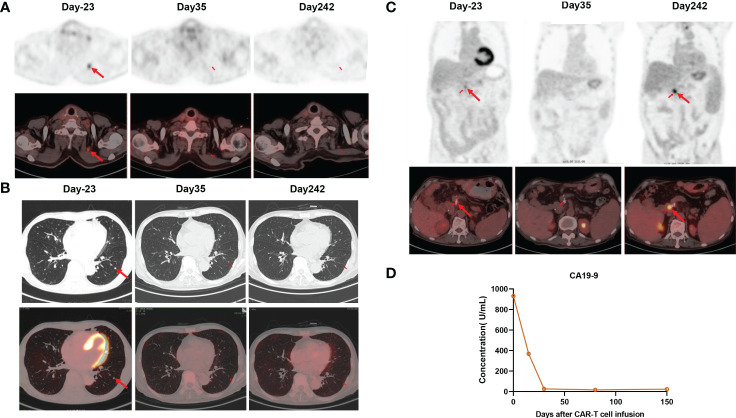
(A) PET/CT showed the disappearance of cervical lymph node metastasis 1 month after claudin18.2-targeted CAR-T cell therapy, and it remained in remission 8 months after CAR-T cell infusion. (B) PET/CT showed the disappearance of lung metastasis 1 month after claudin18.2-targeted CAR-T cell therapy, and it remained in remission 8 months after CAR-T cell infusion. (C) PET/CT showed tumor recurrence at the surgical resection site 8 months after CAR-T cell infusion (D) The levels of CA19-9 were markedly decreased after CAR-T cell infusion.
